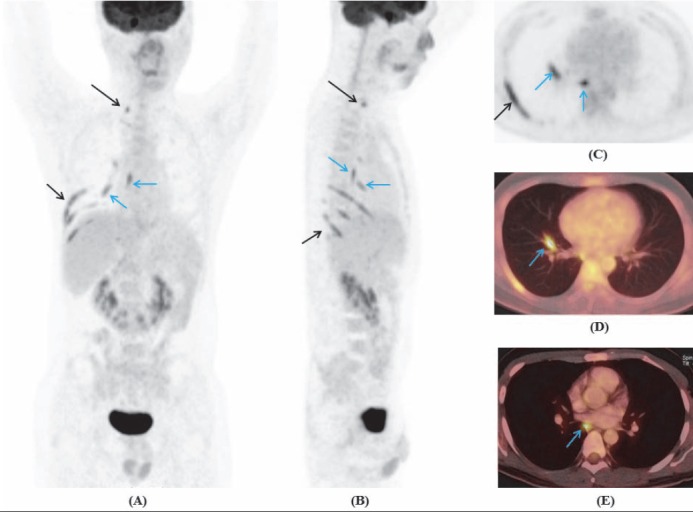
Maximum intensity projection of whole body coronal (A), sagital (B), thoracic transaxial (C), and PET/CT images (D, E) showed increased FDG uptake of the right cervicothoracic ganglion (long arrow), along the 7^th^-10^th^ intercostal nerves, branches of the vagus nerve (blue arrows)
